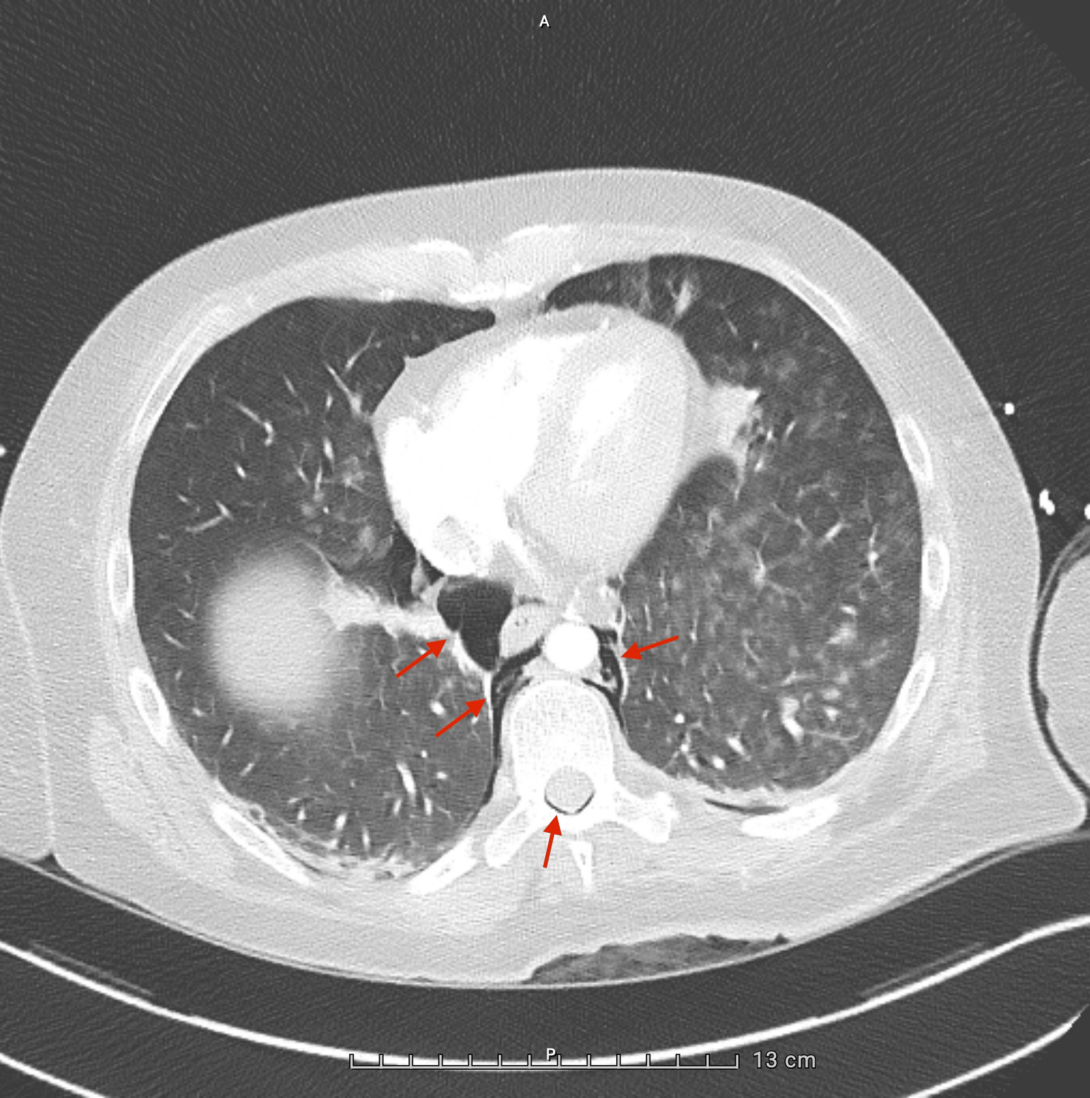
Axial chest CT – lower thoracic level slice showing pneumomediastinum and epidural air. Also showing multiple areas of ill-defined pulmonary opacities, predominantly in the left lung. These findings are consistent with bilateral alveolar filling, most likely of infectious origin.
